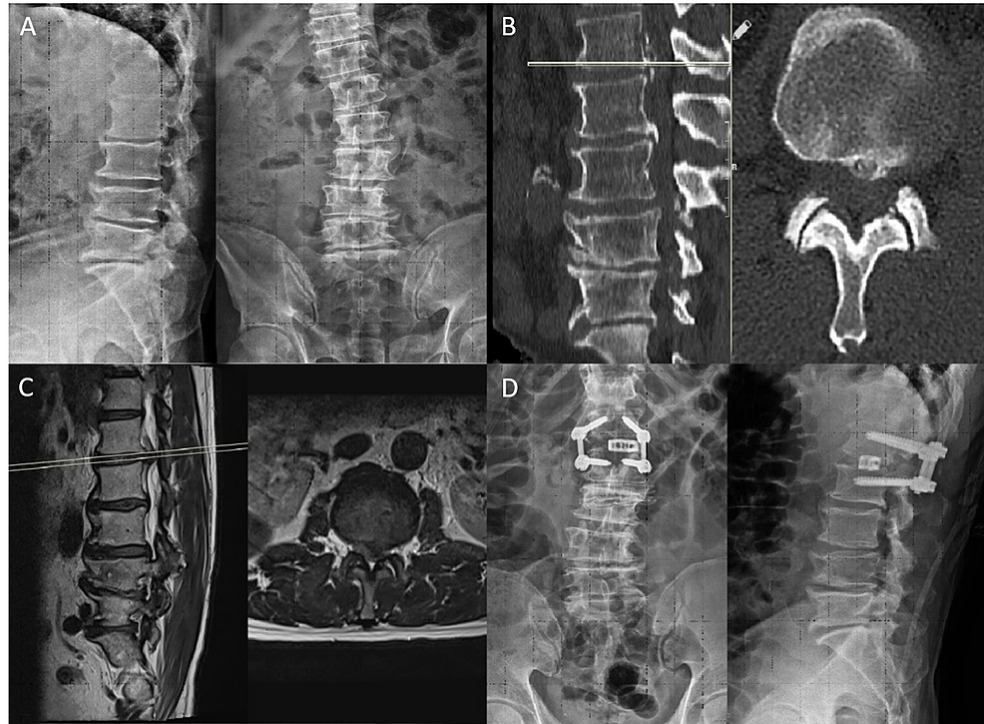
A: Preoperative Two-Way X-ray images, B: Preoperative CT, C: Preoperative MRI, D: Postoperative Two-Way X-ray Images
